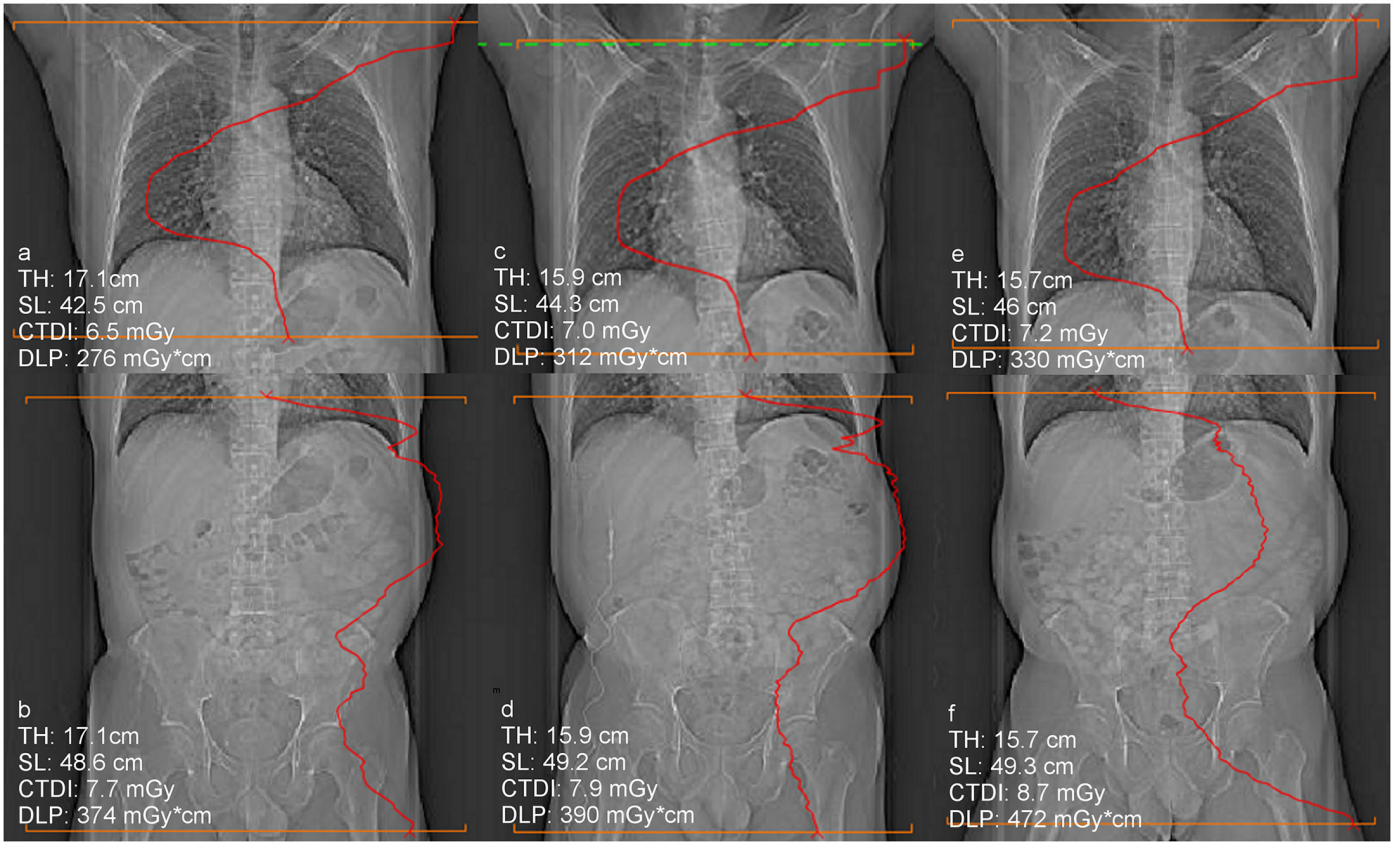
a-f: Scout views of three follow-up CT examinations of a 54 year old male patient with superimposed modulation of the tube current. While the differences in the radiation dose between the chest CT examinations can be explained by differences in the table height (TH) and the scan length (SL), the difference in dose between the abdominal CT scans d and f, cannot be explained by these factors due to the fact that in d and f the table height as well as the scan length had been virtually the same. However, the positioning of the thighs was different between these examinations, which resulted in a different tube current modulation and consecutively in different radiation dose.
